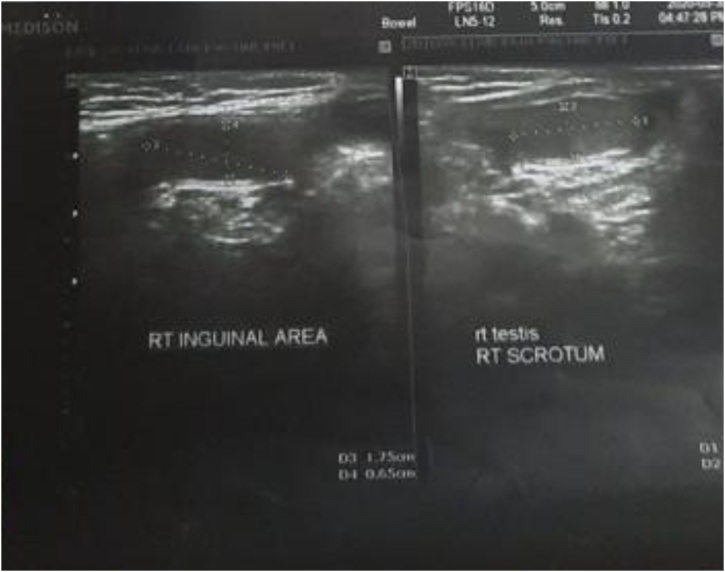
A sonographic picture of the scrotum and the inguinal region showing right testis in the scrotum and left testis in the right inguinal region.
